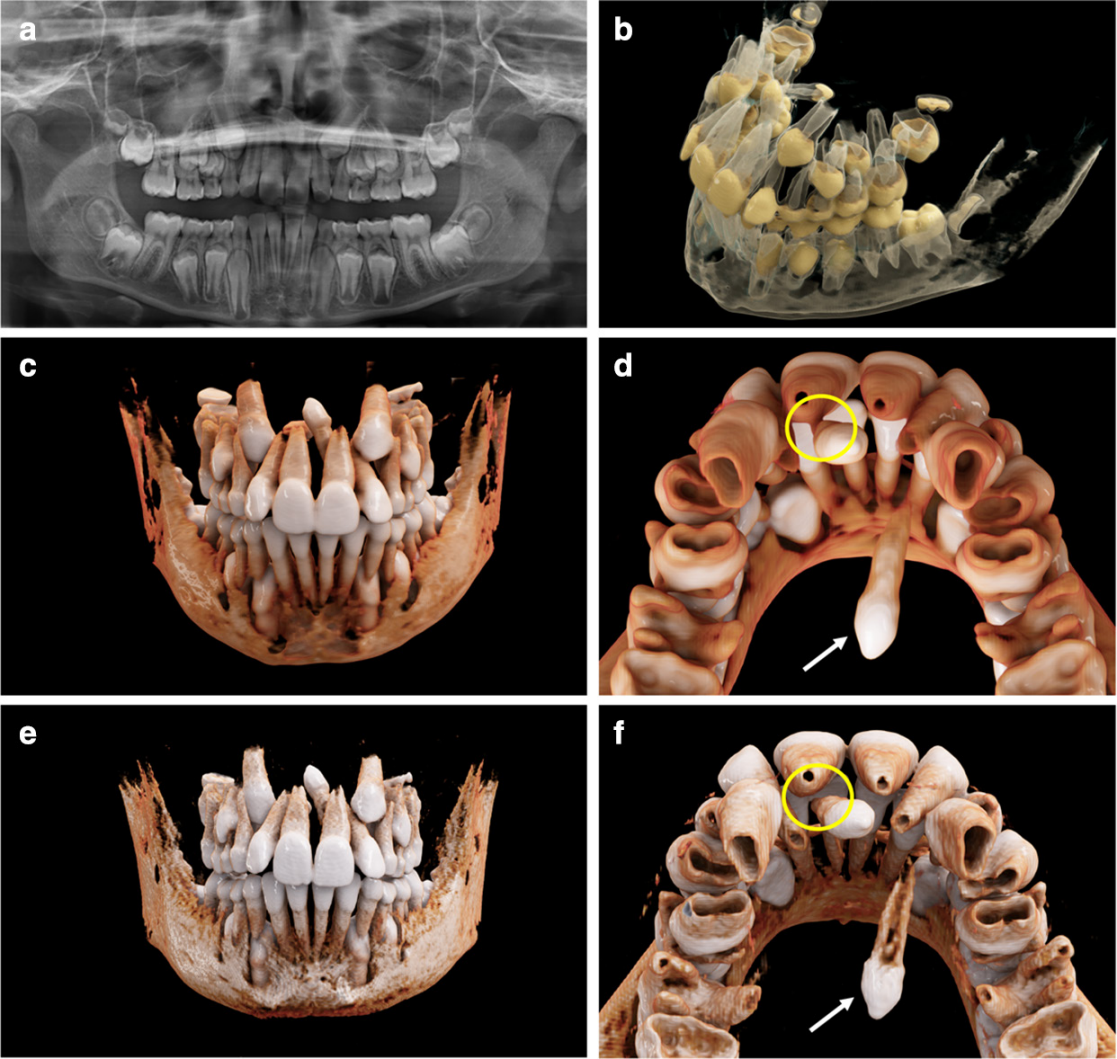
An 11.2-year-old boy with a mesiodens and a further supplementary tooth (white arrows; 3D, f) within the hard palate. (a) A panoramic radiograph is displayed. (b) Semi-transparent reconstruction parameters are displayed. (c, d) The bone reconstruction parameters with a soft kernel can lead to artifacts in regions with teeth near each other (yellow circle, in d). (e, f) The same situation is shown with a hard kernel, allowing a better differentiation between the roots of the mesiodens and of tooth 21 (yellow circle in f).
